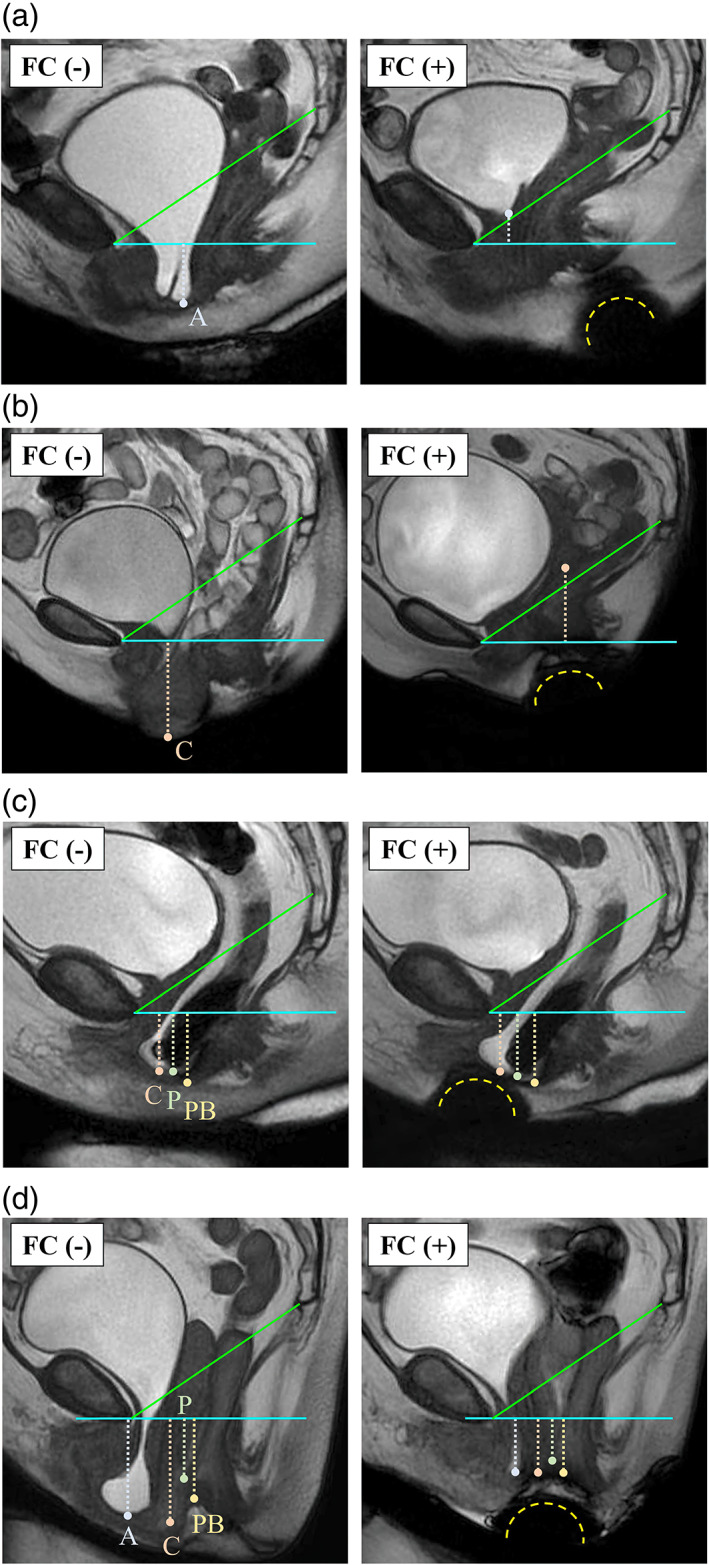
Examples of MRI measurements. The images depict (a) cystocele, (b) uterine prolapse, (c) enterocele and rectocele, and (d) complete eversion. The cushion was identified as a low‐intensity hemisphere below the perineum (yellow‐dashed lines)
